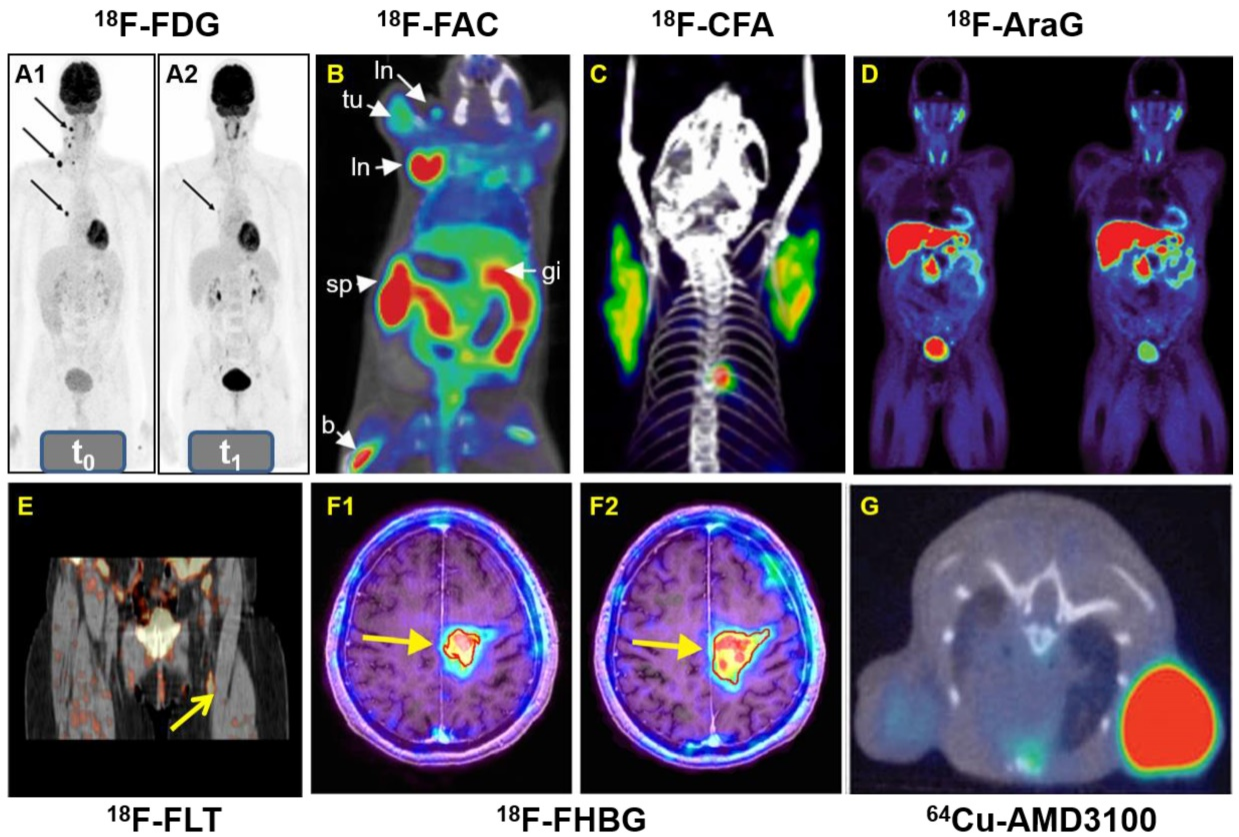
A: Whole-body ^18^F-FDG-PET examination at two time points: before PD-1-therapy start (t_0_, base-line) (A1) and two weeks (t_1_, study examination) (A2). B: ^18^F-FAC was used to evaluate lymphoid organs and immune activation, tu: tumor; ln: lymph node; sp: spleen; gi: gastrointestinal tract; b: bone. C: Detection of immune responses after immunotherapy in glioblastoma using ^18^F-CFA. D: Pharmacokinetics of ^18^F-AraG in a healthy human volunteer to prepare to visualize activated T cells in acute graft-versus-host disease. E: Early identification of antigen-specific immune responses *in vivo* by^18^F-FLT. F: Reporter gene imaging of targeted T-cell immunotherapy in recurrent glioma by ^18^F-FHBG, F1: Pre-immunotherapy using CD8^+^ cytotoxic T lymphocytes (CTLs), F2: Post-immunotherapy using CD8^+^ CTLs. G: ^64^Cu-AMD3100-PET/CT for imaging of CXCR4 expression in subcutaneous brain tumor xenografts.
